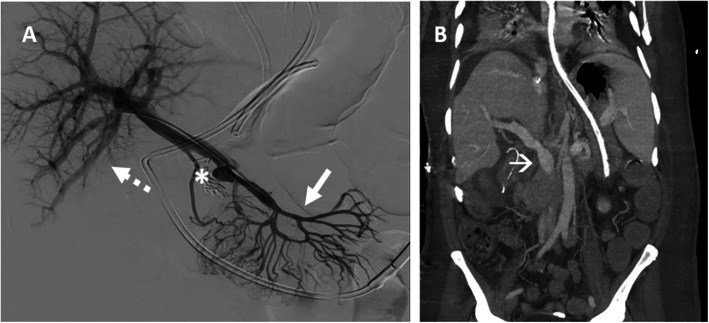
a Patient #1: Portography showing patent mesenteric vein (arrow) with the pseudoaneurysm at the porto-mesenteric confluence (asterisks), with a patent intra-hepatic portal vein system (dotted arrows). b Patient #2: CT scan showing irregularities of the extrahepatic wall of the PV (arrow), on the site of previous bleedings
